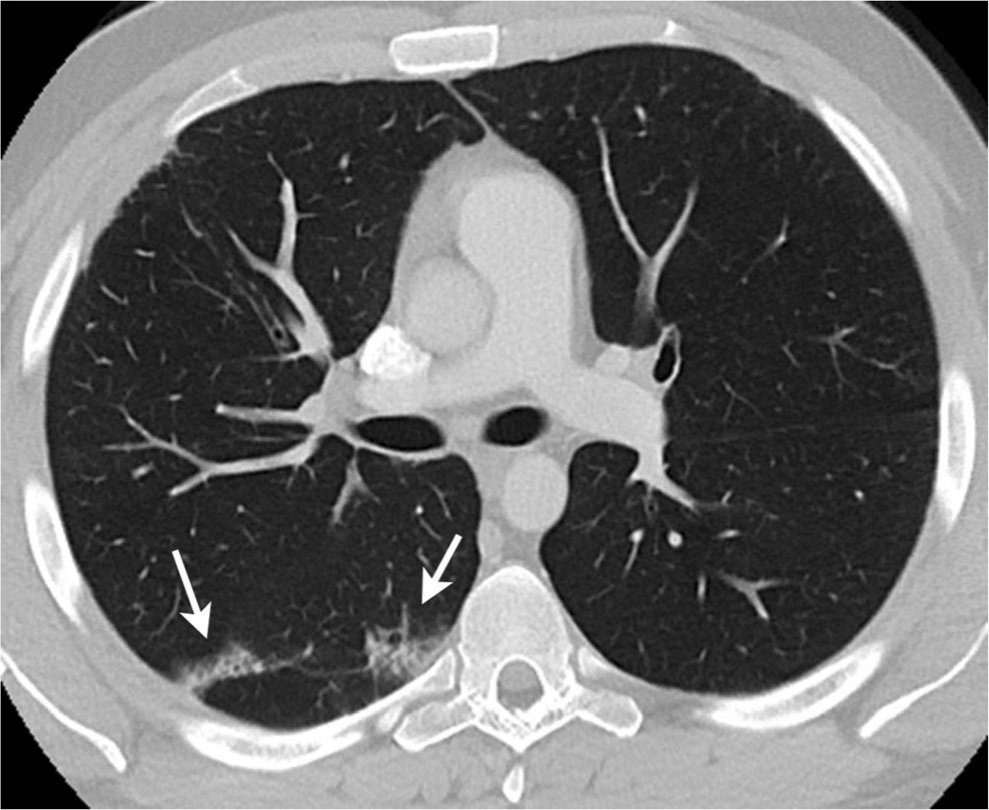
A 22-year-old male with nodular sclerosing HL on treatment with an ABVD regimen and complaining of new onset cough without fever. Axial contrast-enhanced lung window CT image shows patchy ground-glass opacities in the right lower lobe with peripheral subpleural distribution suggestive of drug toxicity
